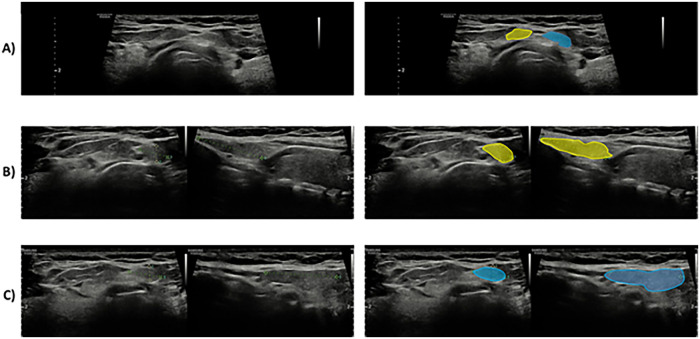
Ultrasound image of thyroid and double pyramidal lobes. In the right part of the image the two pyramidal lobes are highlighted in yellow (right) and blue (left). Panel (A): Antero-posterior view. Panel (B) Longitudinal view of the right pyramidal lobe. Panel (C) Longitudinal view of the left pyramidal lobe.
